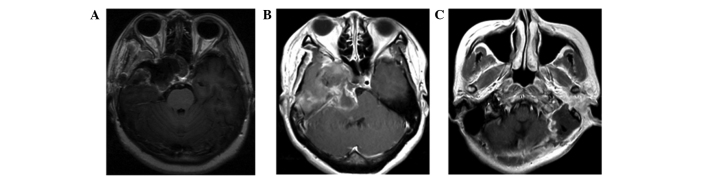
Post-operative MRI scans with contrast showing (A) total and (B and C) near-total tumor resection. MRI, magnetic resonance imaging.
